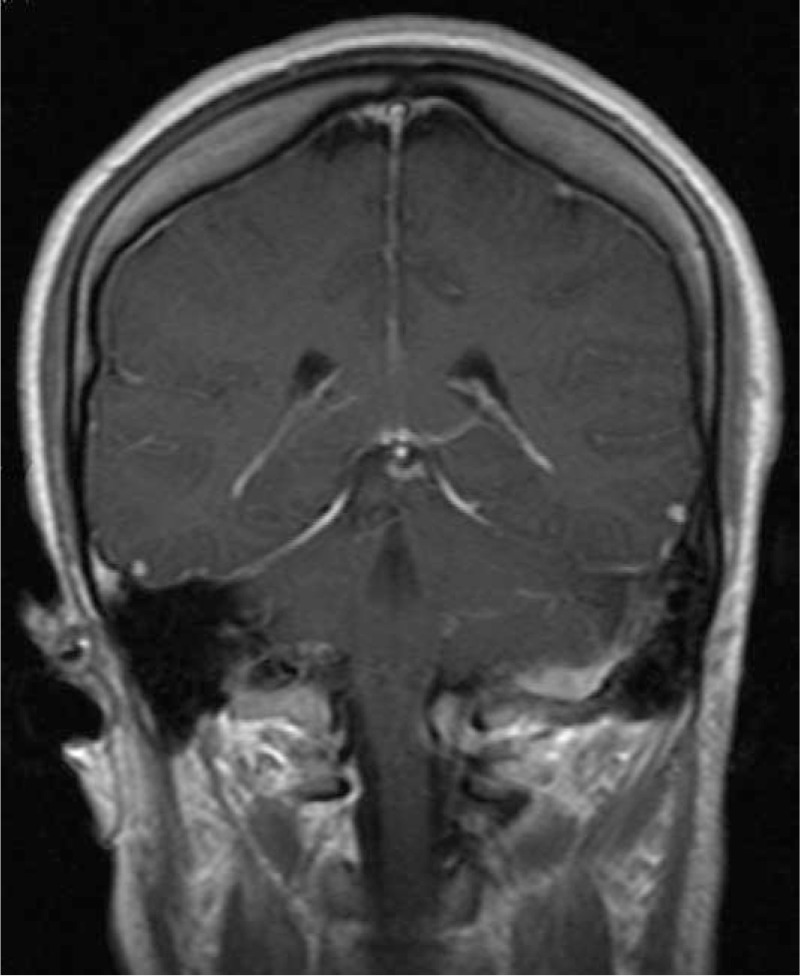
Postoperative MRI scan was performed on the 7th day after the operation revealed the bulk of lesion in the foramen magnum had been removed with little residual lesions.
